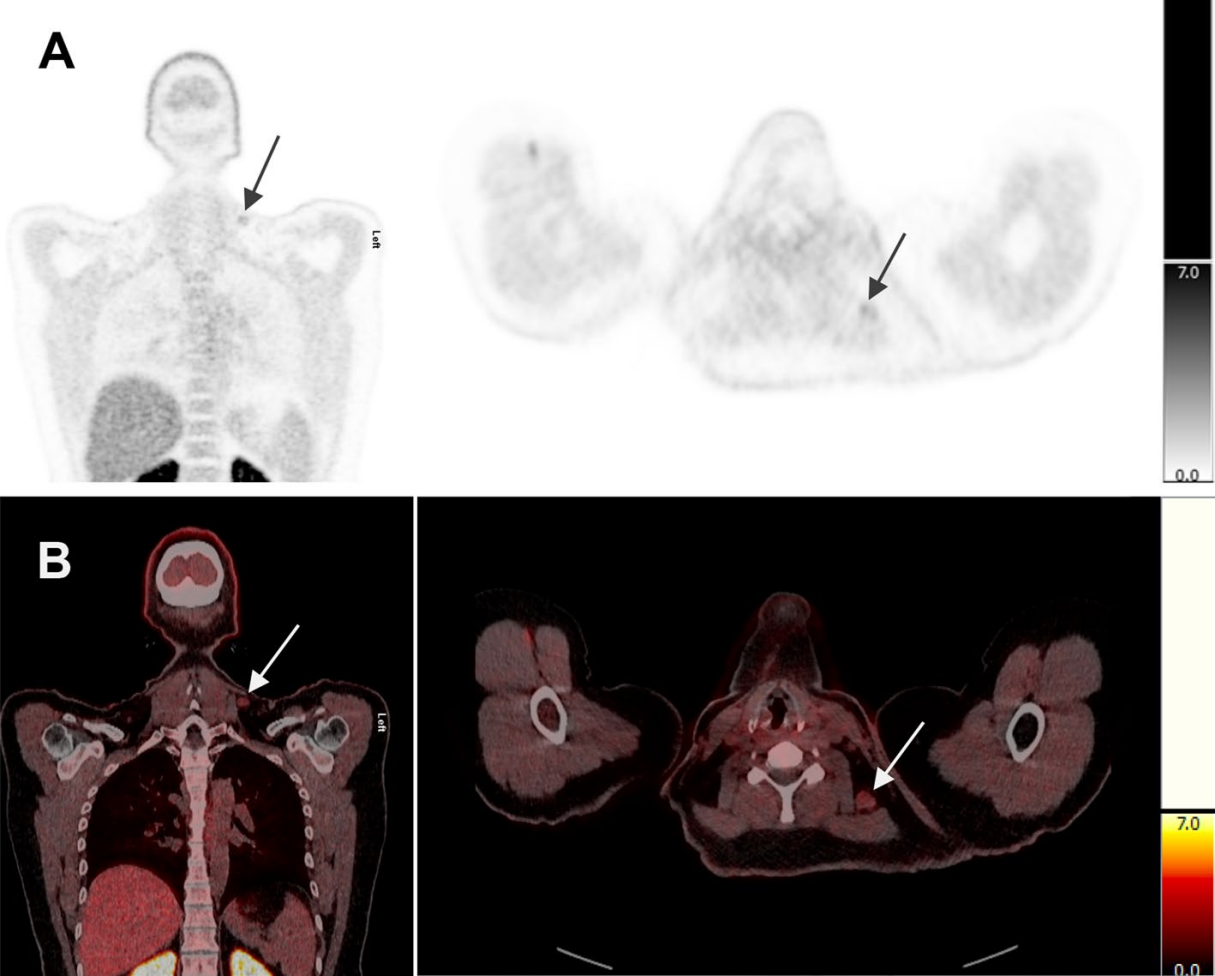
A 40-year-old woman (patient 9). Coronal and axial PET A and fused PET/CT B images showing slightly increased [^18^F]FDOPA uptake in a cervical lymph node (SUV_max_ = 2) on the early acquisition. Subsequent biopsy showed reactive changes and no malignancy
